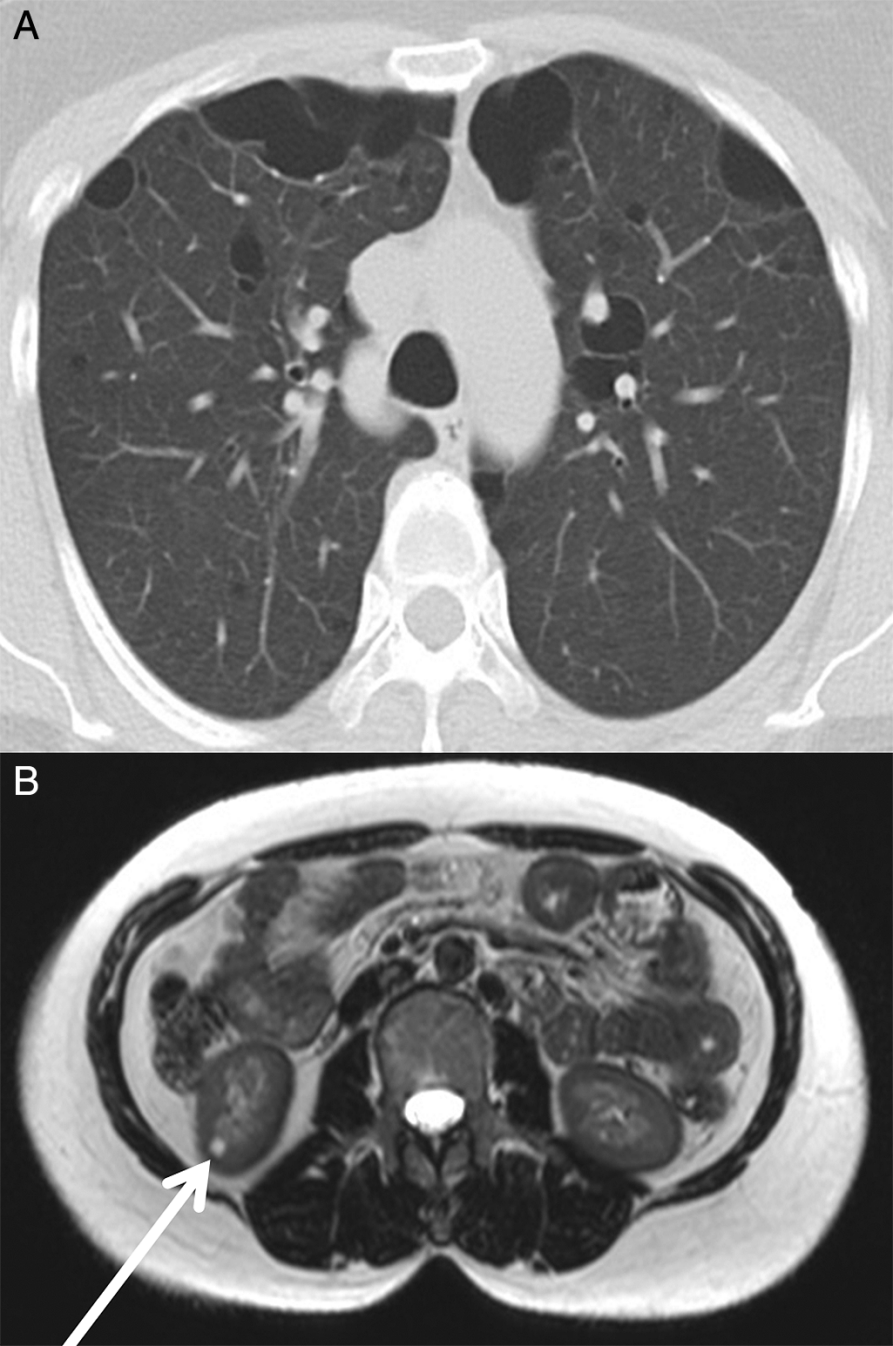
(A) Thoracic computed tomography of the patient showed multiple bilateral pulmonary cysts. (B) Abdominal magnetic resonance imaging revealed a renal cyst (white arrow).
